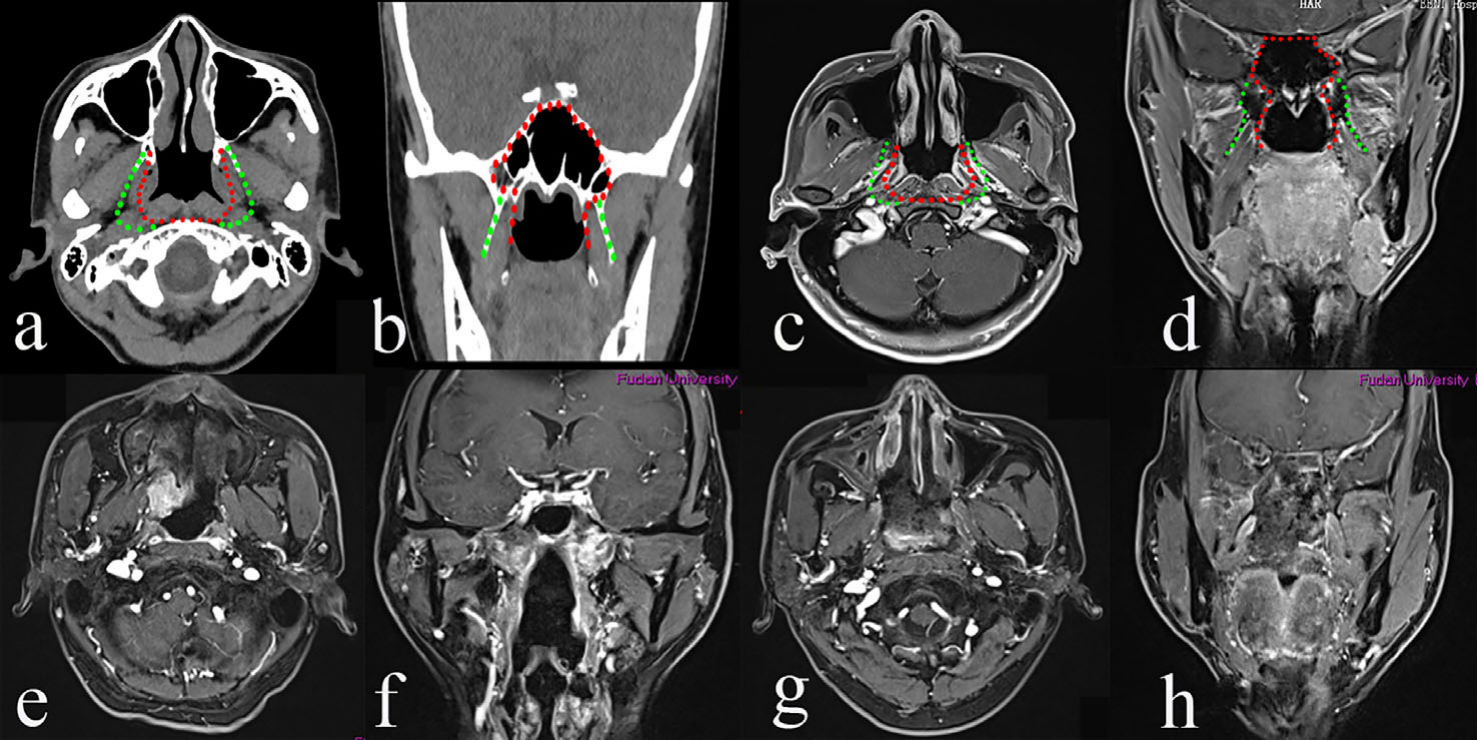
The greatest extent of resection in Type II transnasal endoscopic nasopharyngectomy (TEN) [green dot line in (a–d)], pre-operative magnetic resonance imaging (MRI) of rT2 nasopharyngeal carcinoma (NPC) (e, f), and post-operative MRI (g, h).
